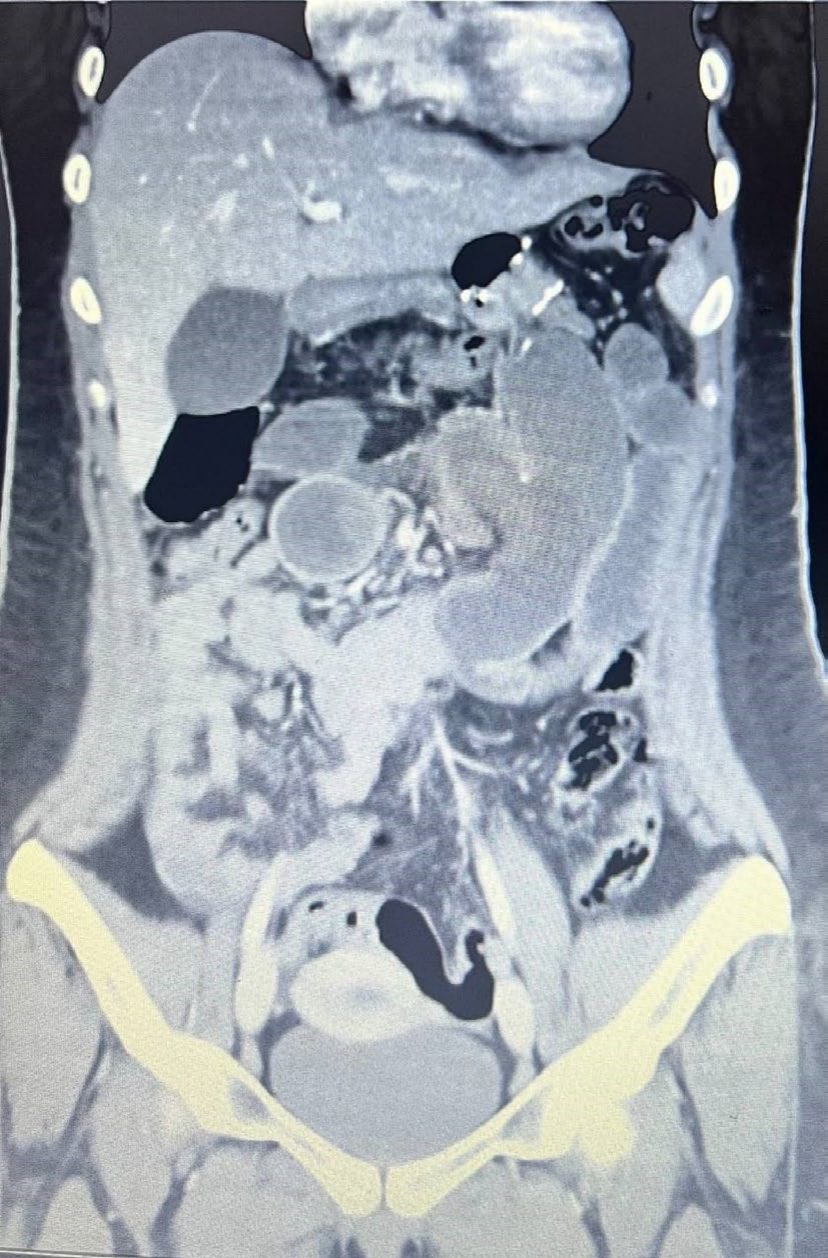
Abdominal CT demonstrating the target sign of a small‐bowel intussusception.
